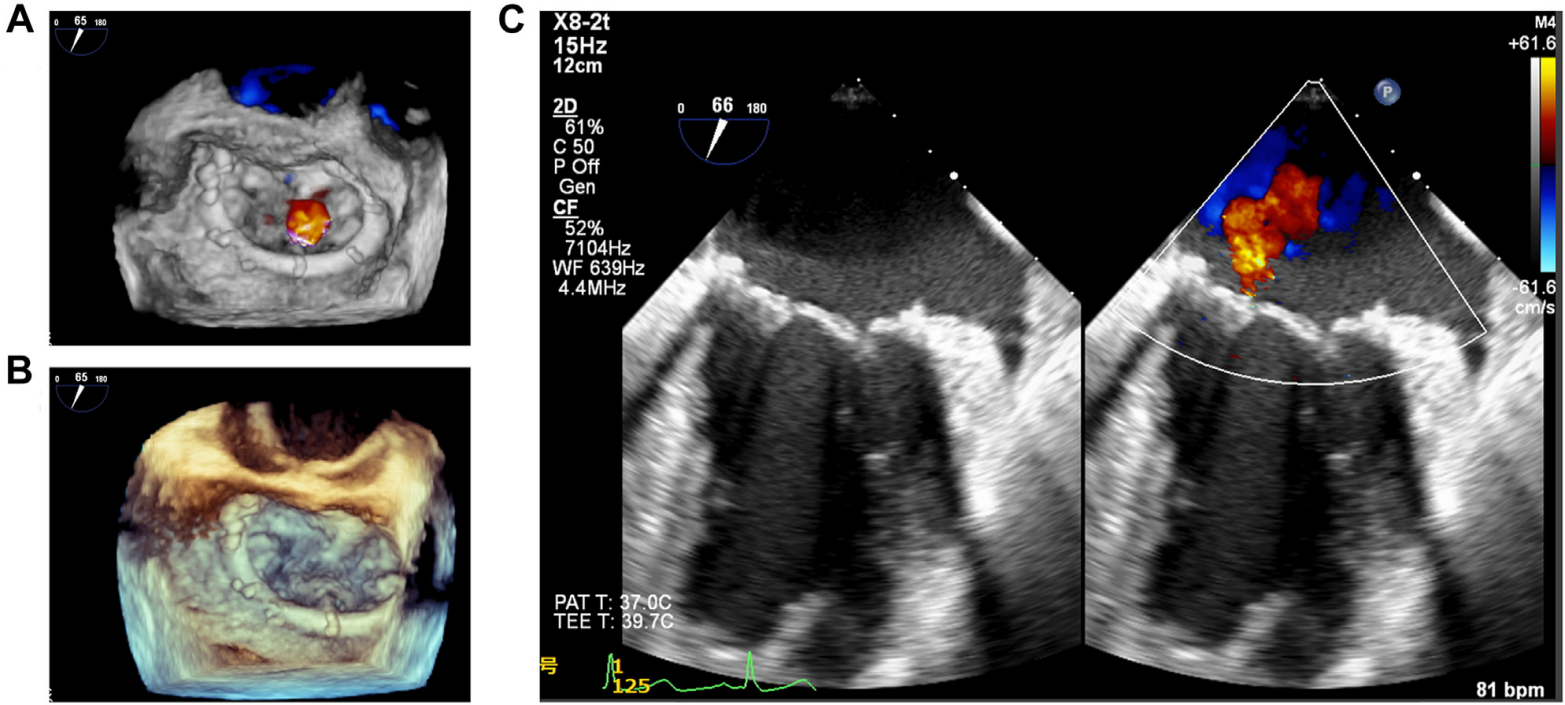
Intraoperative transesophageal echocardiography findings after mitral valve repair. (A, B) Three-dimensional constructed mitral valve images. (C) Midesophageal mitral commissural view. After the repair with chordal plasty and ring annuloplasty, the mitral valve shape is corrected, but a central jet corresponding to mild regurgitation is revealed at the indentation between P2 and P3.
